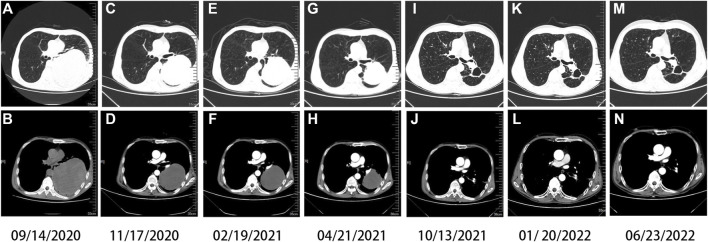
Chest computed tomography (CT). Imaging findings during the patient’s course (A,B) at baseline (solid tumor, dmax = 13.2 cm × 11.1 cm), (C,D) after one cycle of Tislelizumab and two cycles of chemotherapy (solid tumor, dmax = 11 cm × 8.7 cm), (E,F) after three cycles of Tislelizumab (solid tumor, dmax = 9.5 cm × 8.1 cm), (G,H) after five cycles of Tislelizumab (solid tumor, dmax = 7.4 cm × 7.0 cm), (I,J) after ten cycles of Tislelizumab (cavity, dmax = 3.9 cm × 3.2 cm), and (K,L) after thirteen cycles of Tislelizumab (cavity, dmax = 3.6 cm × 2.8 cm); (M,N) in recent recheck (cavity, dmax = 3.3 cm × 2.5 cm), Anlotinib is in continuous use.
